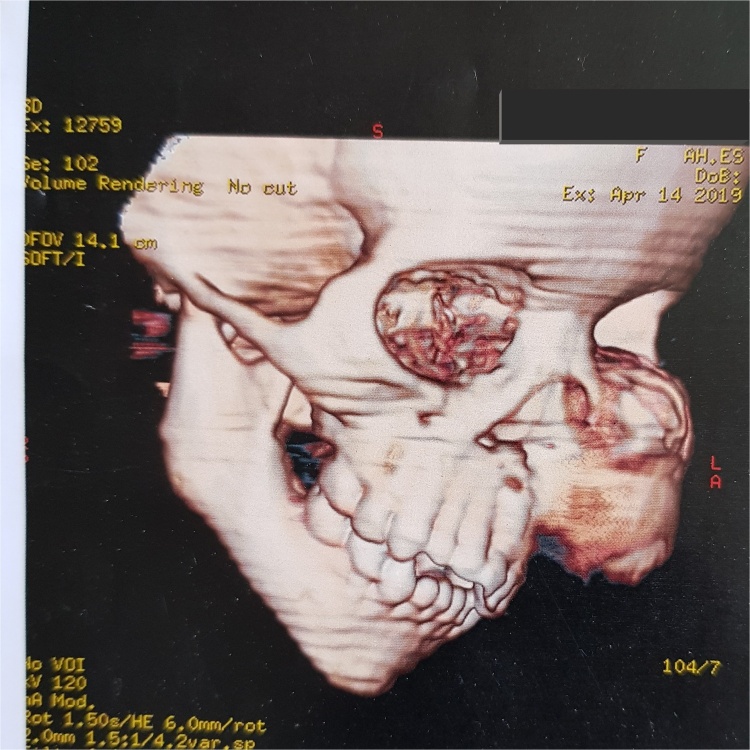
3D volume reconstructed CT showing the destruction and extension of the tumor into the left orbit.
